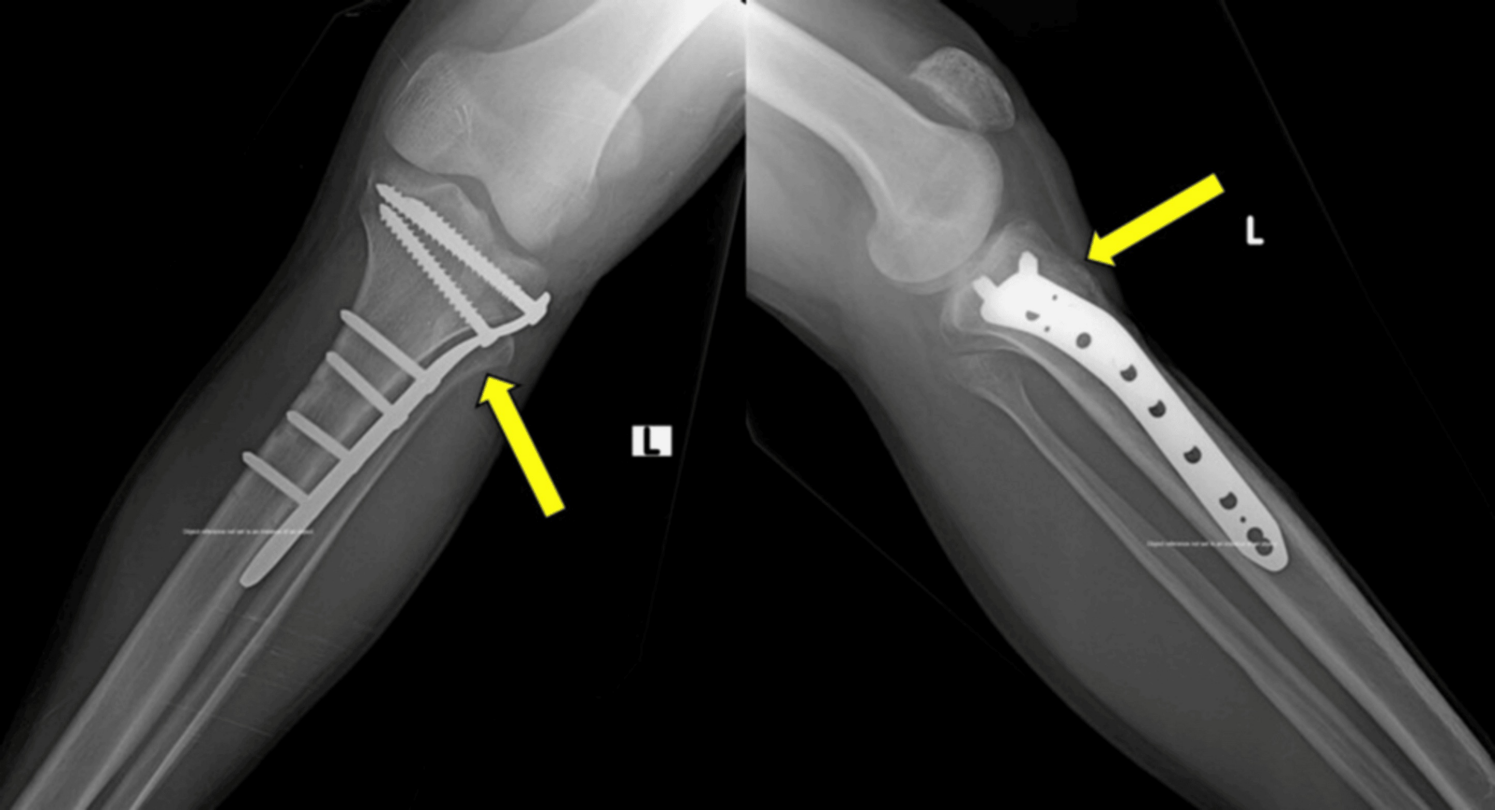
Follow-up radiographs at six months demonstrating fracture union with maintained alignment.
